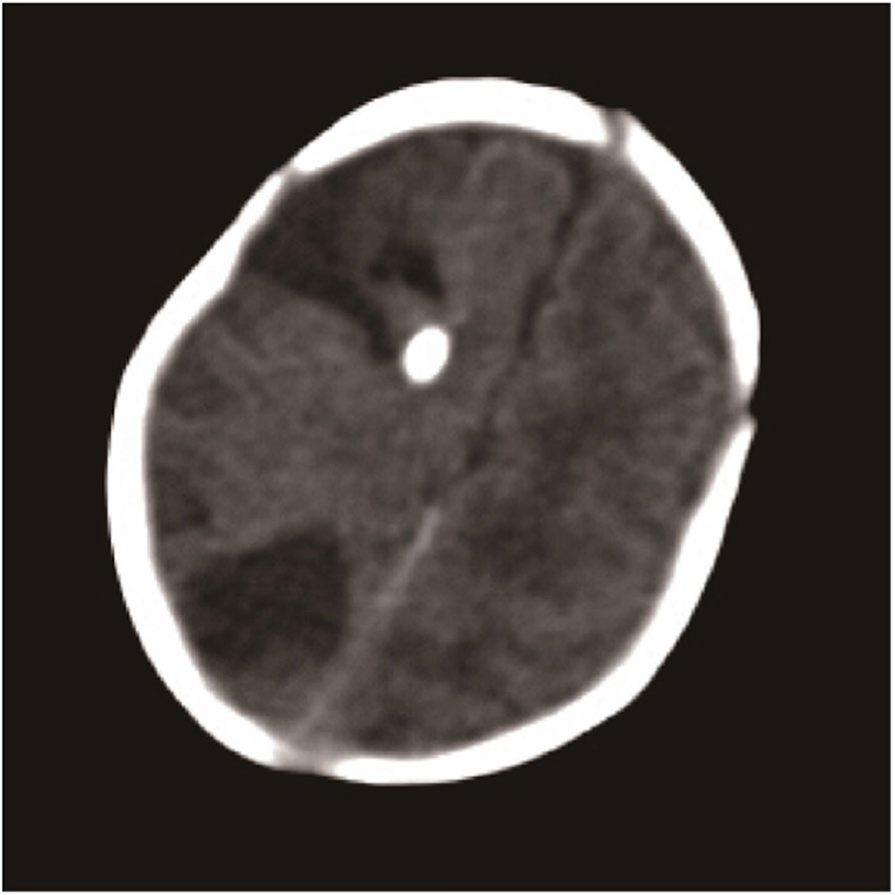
CT showed a decreased size of abscess in the frontal lobe and the hyperintensity area showed as an external ventricular drain. In the parietal area, there was an abscess with decreased attenuation. (Clarity of the figure was limited by the digital technology in our hospital in 2001)
